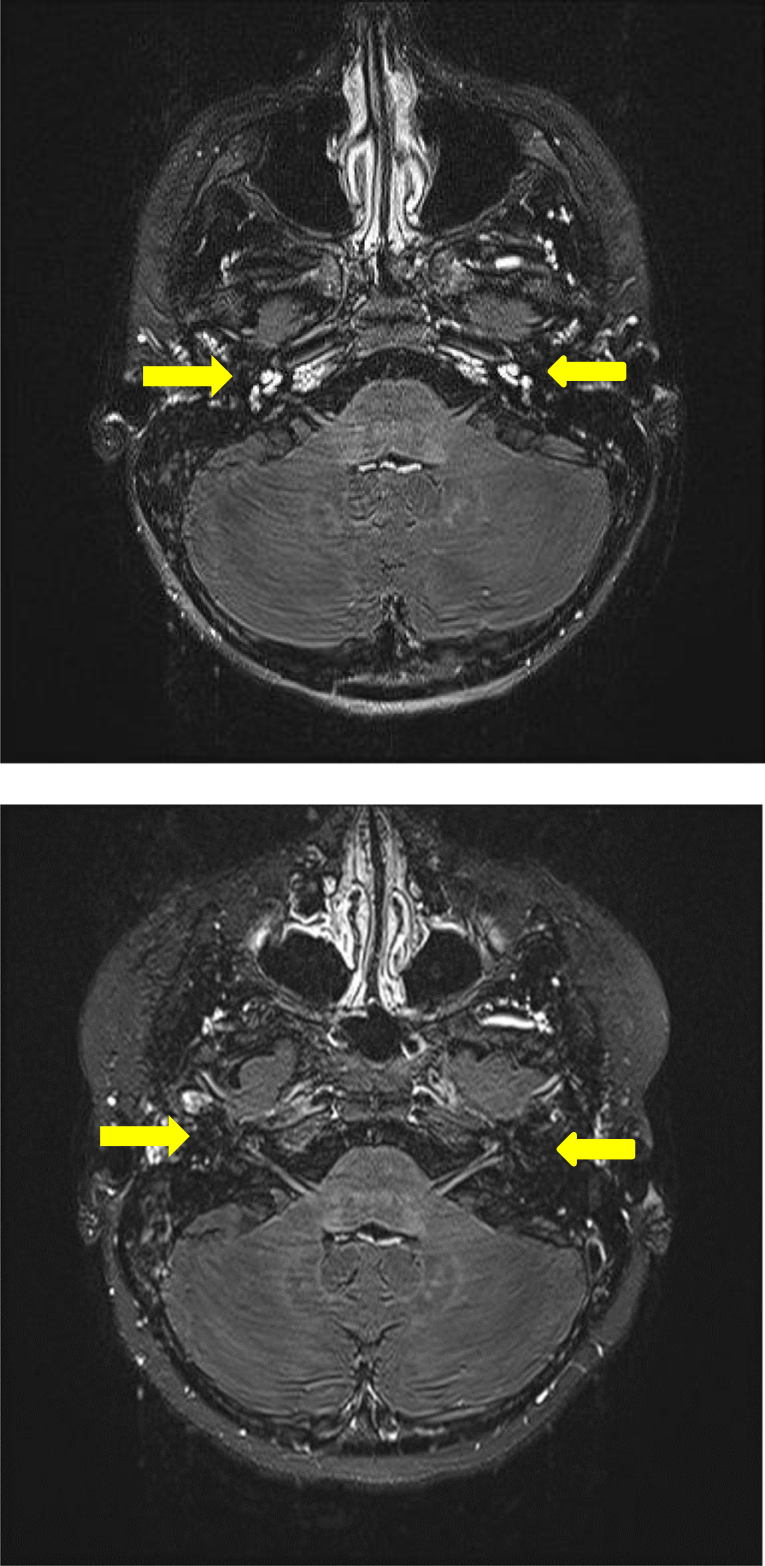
MR images in STIR sequences showing a) florid labyrinthitis before treatment and b) absence of labyrinthitis after 4 months of immunomodulatory treatment
